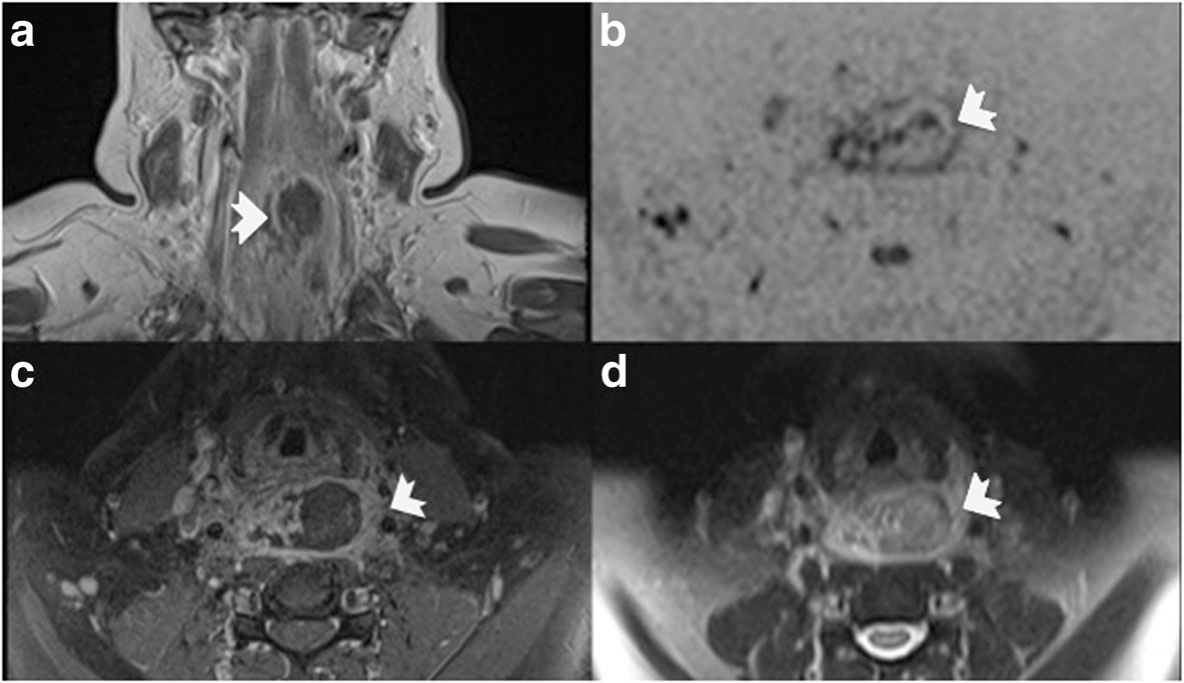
Magnetic resonance imaging of retropharyngeal hematoma. a A lesion with rim enhancement (*arrow*) is displayed on a T1-weighted coronal image. b Diffusion restriction can be found at the margins of the lesion (*arrow*). c and d T1-weighted fat suppressed and T2-weighted images accordingly show a lesion with a central core, surrounded by an enhancing rim. The intermediate hyperintense signal on T2-weighted (*arrow*) image indicates hematoma
